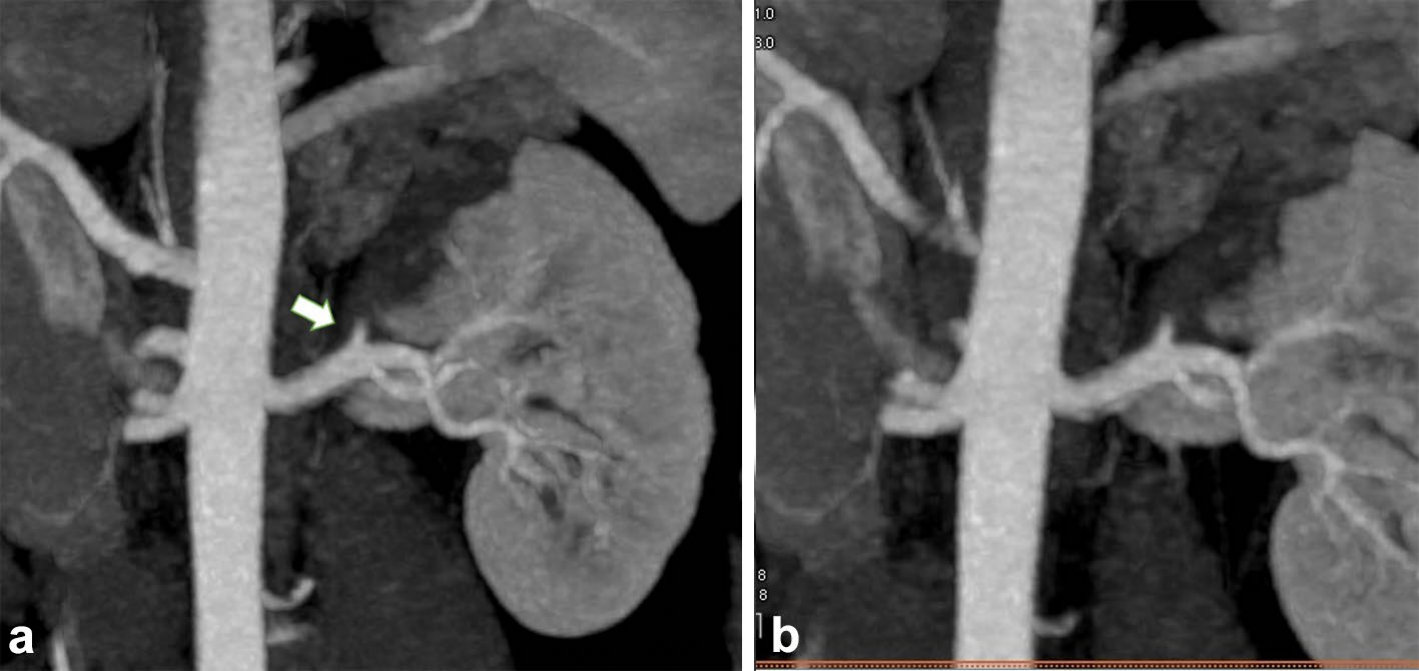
CT coronal A, B views shows a faintly perfused left kidney and thrombotic occlusion of the segmental apical renal artery (white arrow)
